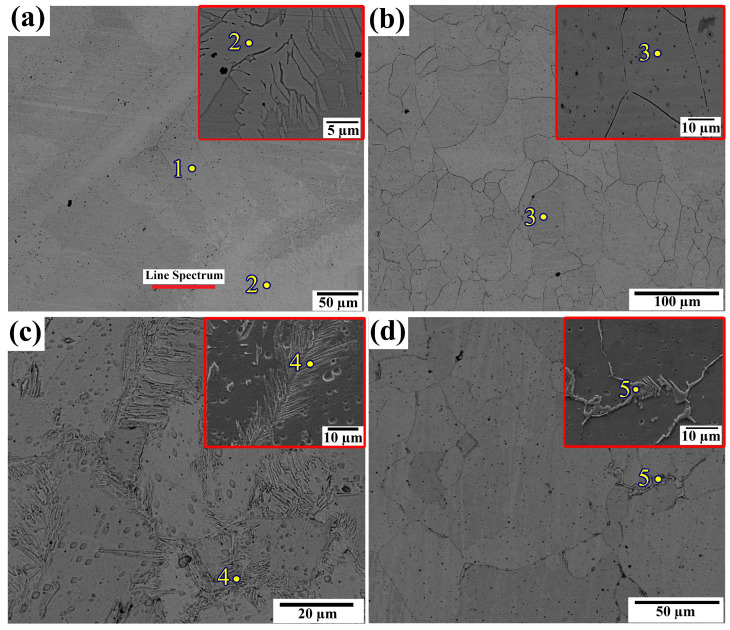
Microstructure of HEA R-UVALC coating: (a) microstructure without ultrasonic vibration with point EDS at 1 and point EDS of the closed subset of point 2, (b) microstructure with ultrasonic vibration amplitude of 5 µm with point EDS at 3 and closed subset of point 3, (c) microstructure with ultrasonic vibration amplitude of 10 µm with point EDS at 4 and closed subset of point 4, and (d) microstructure with ultrasonic vibration amplitude of 15 µm with point EDS at 5 and closed subset of point 5; EDS line spectrum marked as line spectrum inside (a).
